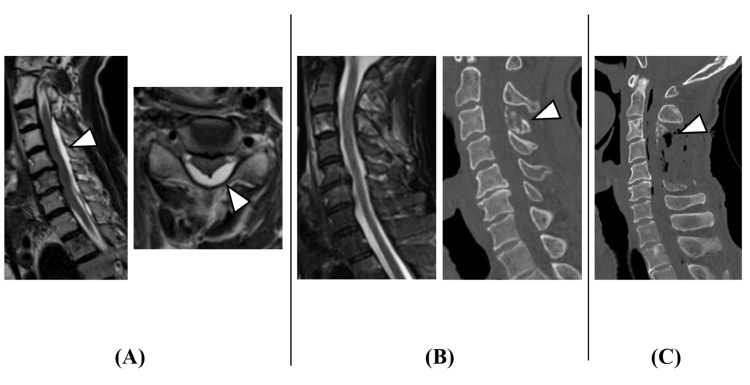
Cervical images (A) Cervical spine MRI at onset (T2-weighted sagittal image and axial image). Due to the acute onset, cervical epidural hematoma was initially suspected (arrowheads). (B) Cervical spine MRI (T2-weighted sagittal image) and CT (sagittal image) obtained two months before symptom onset. There was no obvious epidural effusion. The C3 lamina suggested bone metastasis from lung cancer (arrowhead). (C) Postoperative cervical spine CT (sagittal image). No obvious cervical epidural hematoma was observed intraoperatively. However, serous light yellow effusion was noted. The C3 lamina, which had bone metastasis from lung cancer, was partially resected (arrowhead) CT: computed tomography; MRI: magnetic resonance imaging
